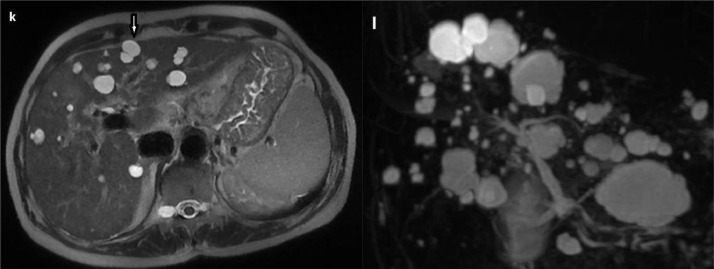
Liver MRI on T2 WS (K) and 3 D MRCP (L)showing multiple hepatic cysts of variable size hyperintense on T2 without communication with the biliary ducts
